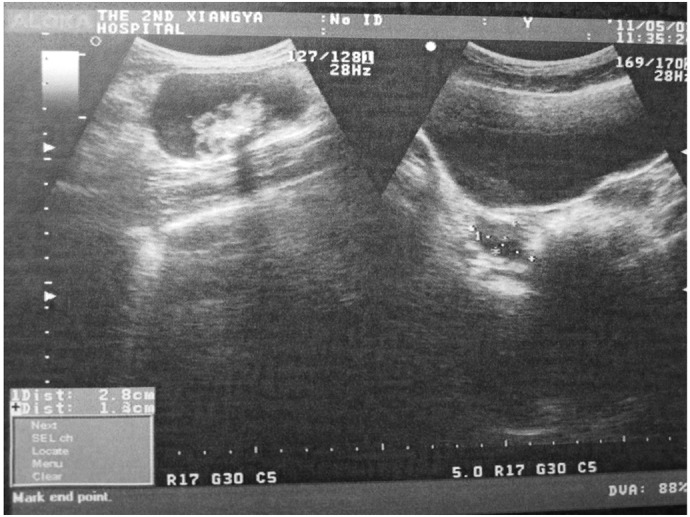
Sonographic examination of the pelvis revealed a cystic mass of ∼8×4 cm in the right hypogastic region.
